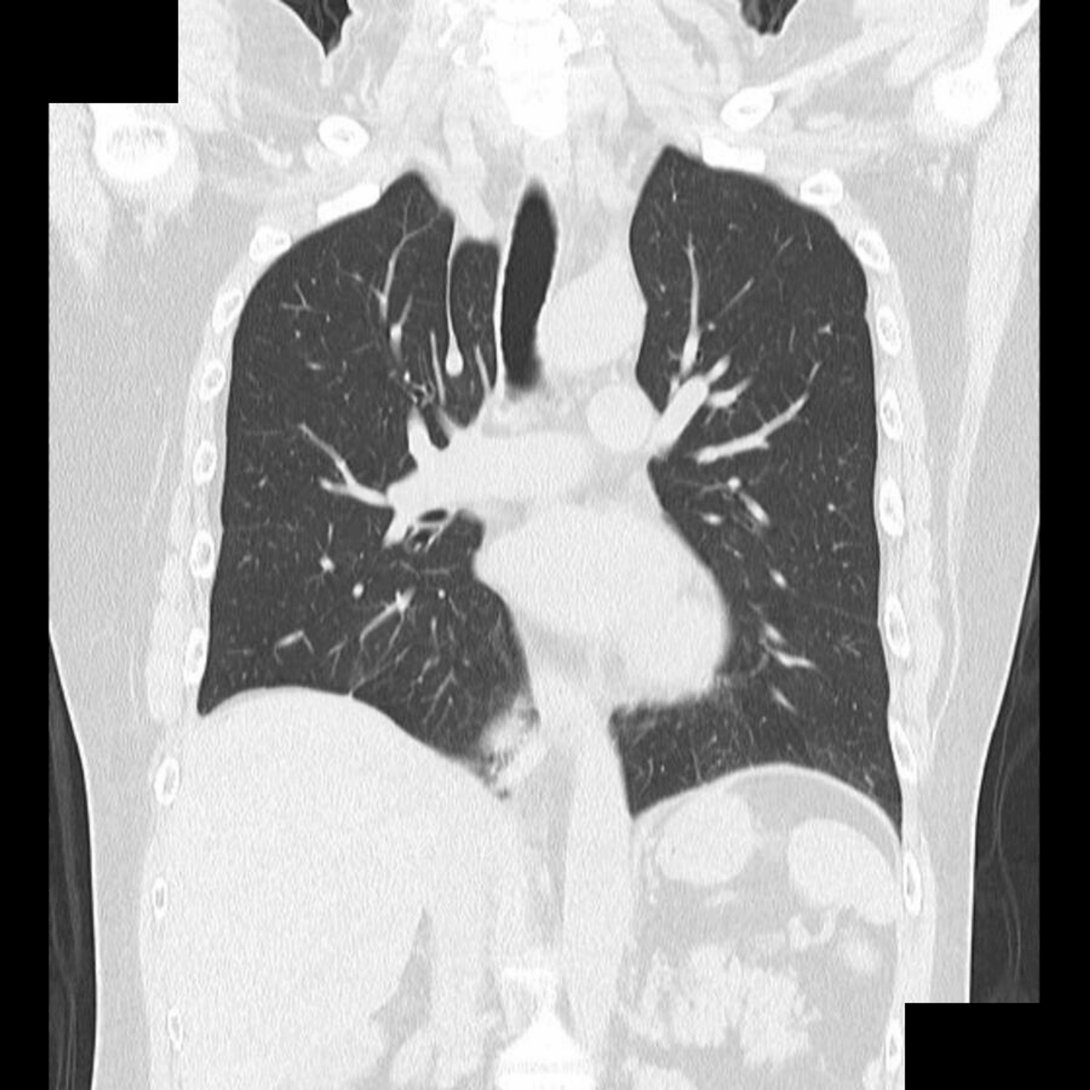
CT showed an abnormal mass in the right lower lobe, which connects to the descending aorta.
